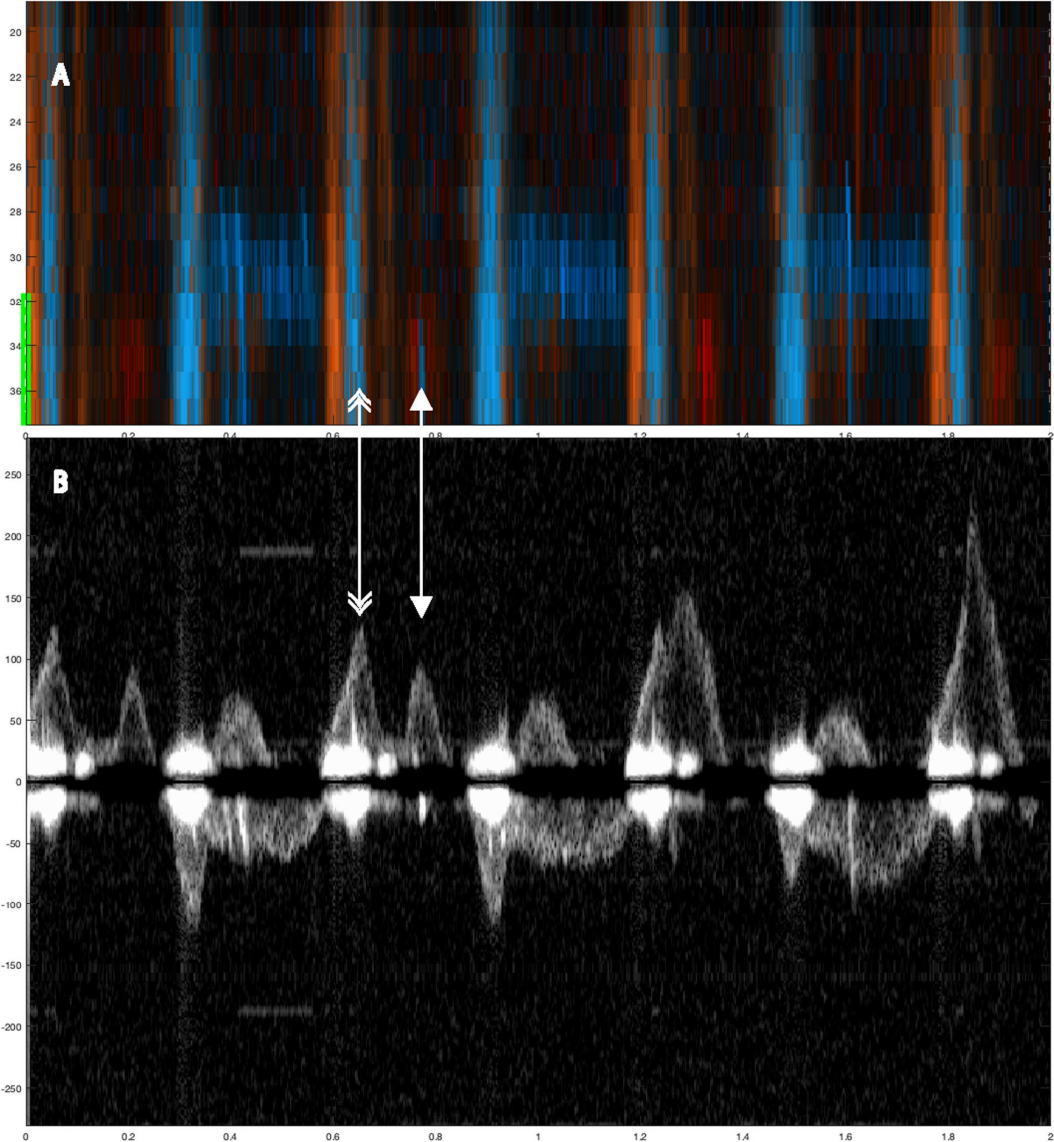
Two-second color M-mode and Doppler Spectrum showing spontaneous circulation during mechanical compression. Color M-mode (A) with the corresponding Doppler specter (B) from the chosen depth below. The figure includes two types of arrows: single-tip arrows indicate spontaneous circulation (S), while double-tip arrows denote chest compressions (C) Velocities are given in cm/s
